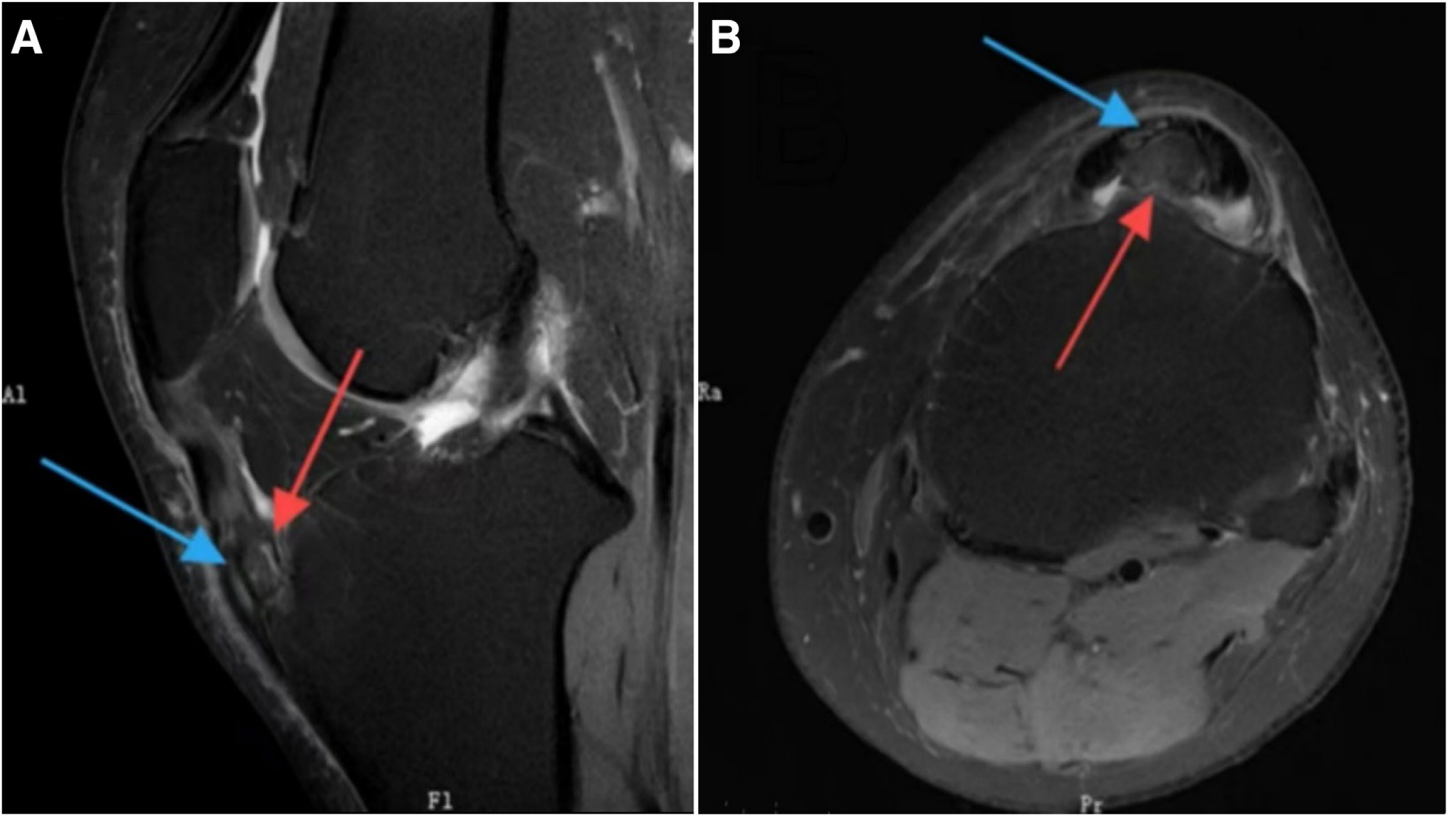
The view of the magnetic resonance imaging of the patient’s left knee. The area pointed by the red arrow is the tibial tuberosity osteophyte, and the area pointed by the blue arrow is damage at the insertion of the patellar tendon. Image A is in the sagittal plane, and image B is in the axial plane.
